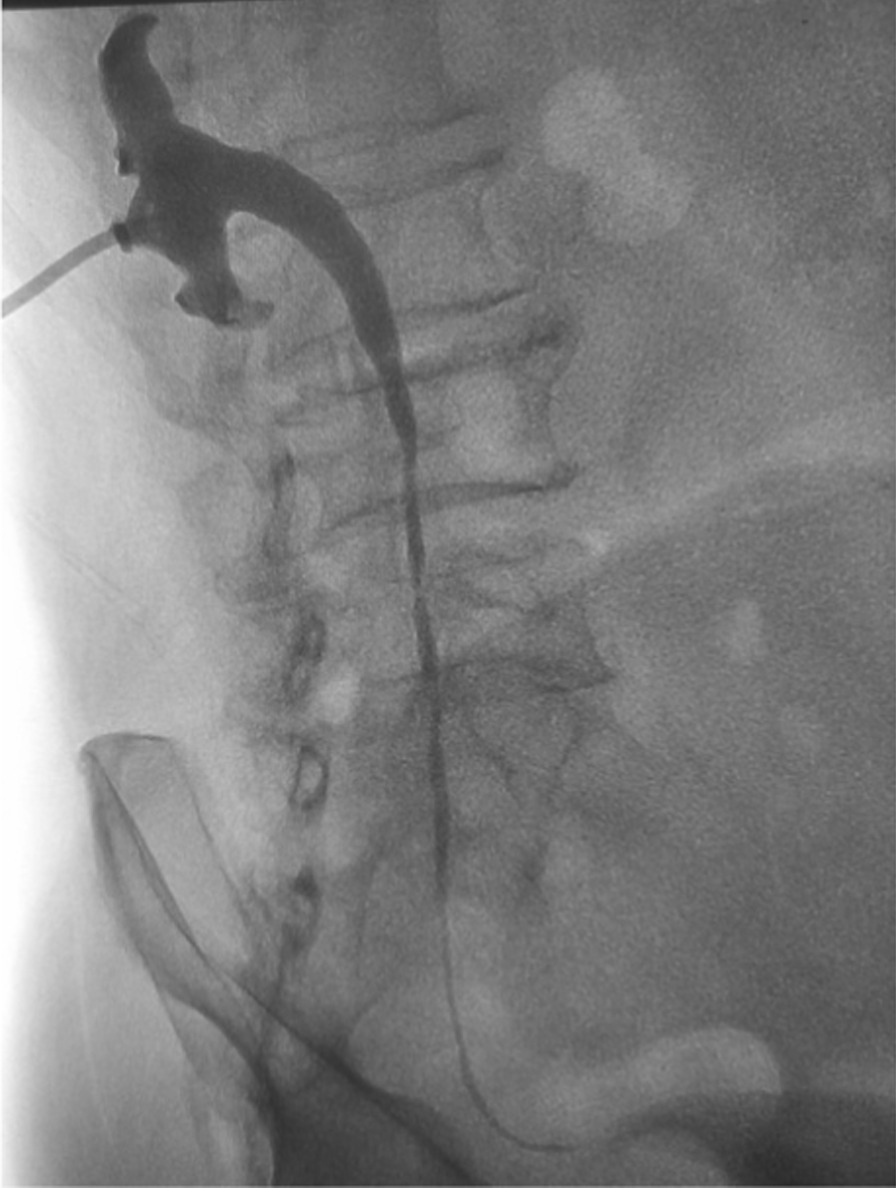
The end of the drainage tube is pulled into the renal pelvis. The contrast medium smoothly enters the bladder cavity through the ureter by drainage tube radiography
